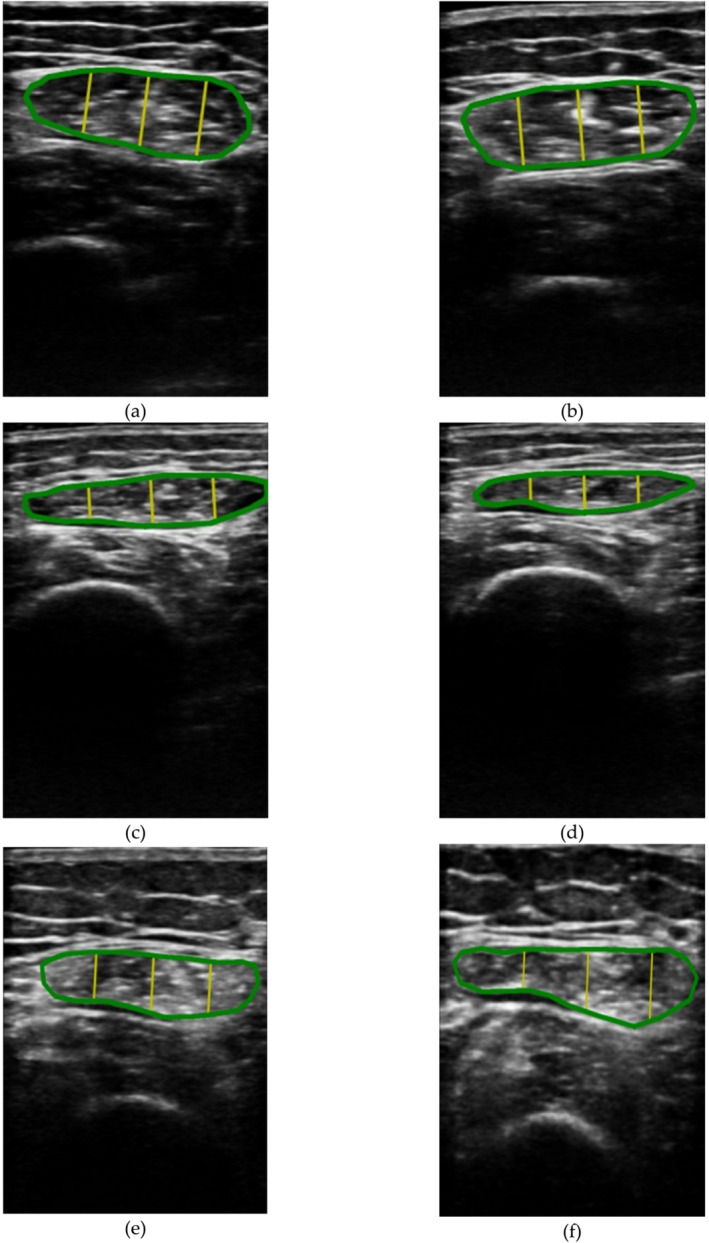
The figure shows an example of three cases of the acquired ultrasound images of the rectus femoris muscle in the transverse plane (automatic segmented ROI of the muscle belly in green colour, and the yellow lines indicate the three measurements of the muscle thickness to compute its mean thickness value). Images (a), (c) and (d) are the acquired images and thickness measurement previous the treatment with the ONS enriched with HMB. The images (b), (d) and (f) are the acquisition and thickness measurements, of the same patients, post treatment with the ONS enriched with HMB. The average of the three yellow marks of (d)–(f) were superior to (a)–(c), respectively.
